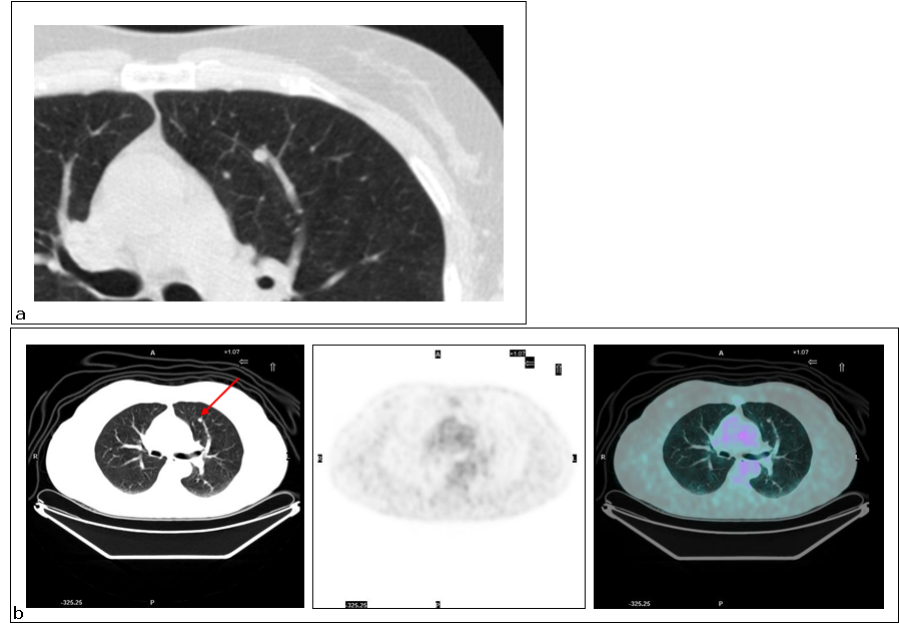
Lung metastasis. Patient #11. Lung metastasis in the upper left lobe. (a) CT interpreted it as benign (FN CT) as did (b) PET (FN PET/CT).
